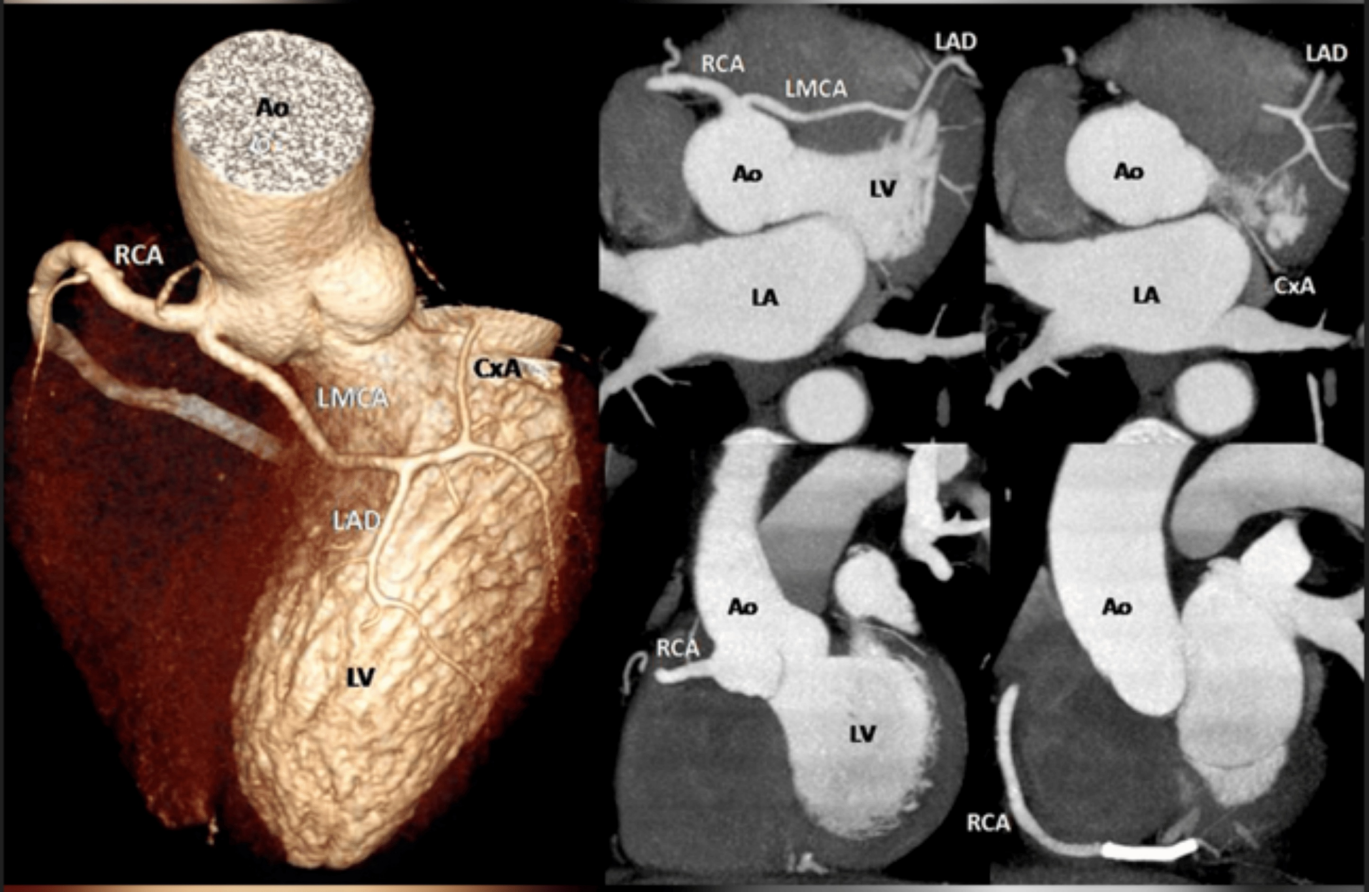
Coronary CT angiography Anomaly of the LMCA that emerges from the RCA with an intraseptal course directed to the middle one-third of the atrioventricular groove, where emerges the distal middle segment of the left anterior interventricular branch, a diagonal branch, and a thin ascending branch that corresponds to the circumflex artery. The stent placed in the distal middle third of the RCA is patent. Ao: aorta, CxA: circumflex artery, LA: left atrium, LAD: left anterior descending artery, LMCA: Left main coronary artery, LV: left ventricle, RCA: right coronary artery
